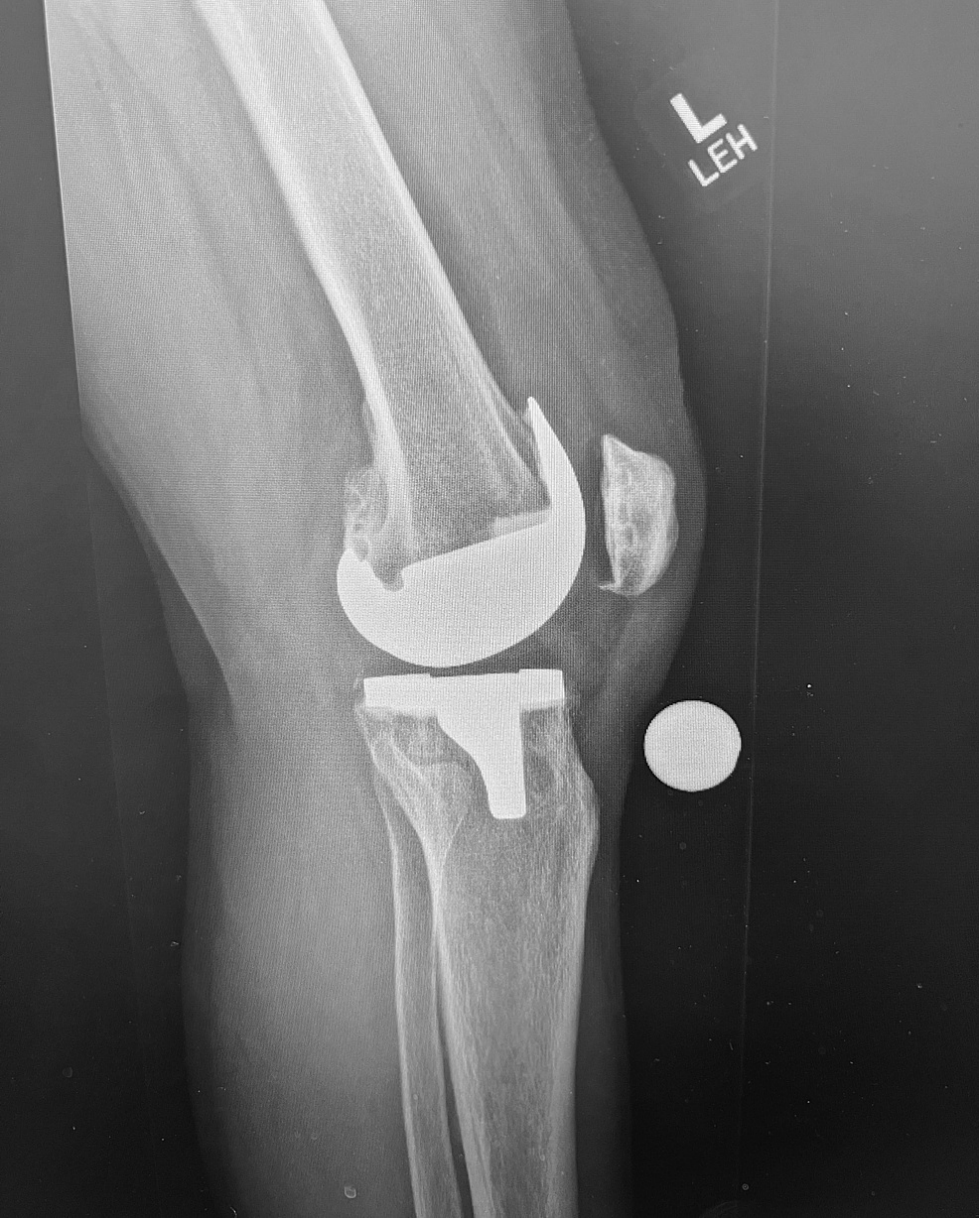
Left lateral knee x-ray demonstrating total knee arthroplasty with mild joint effusion five days post initial joint aspiration.
